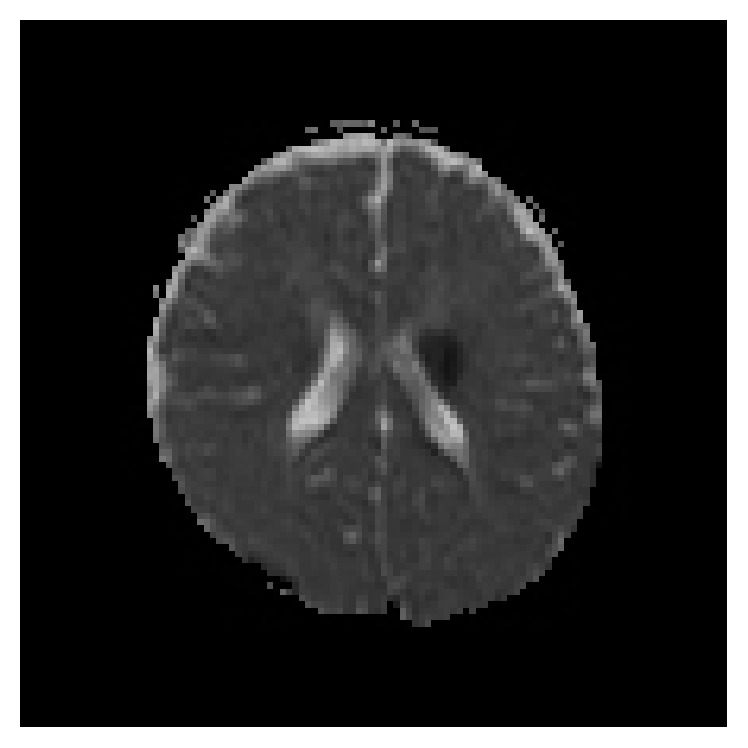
MRI brain (ADC) low signals noted in the left capsule-ganglionic region suggestive of acute infarct.
